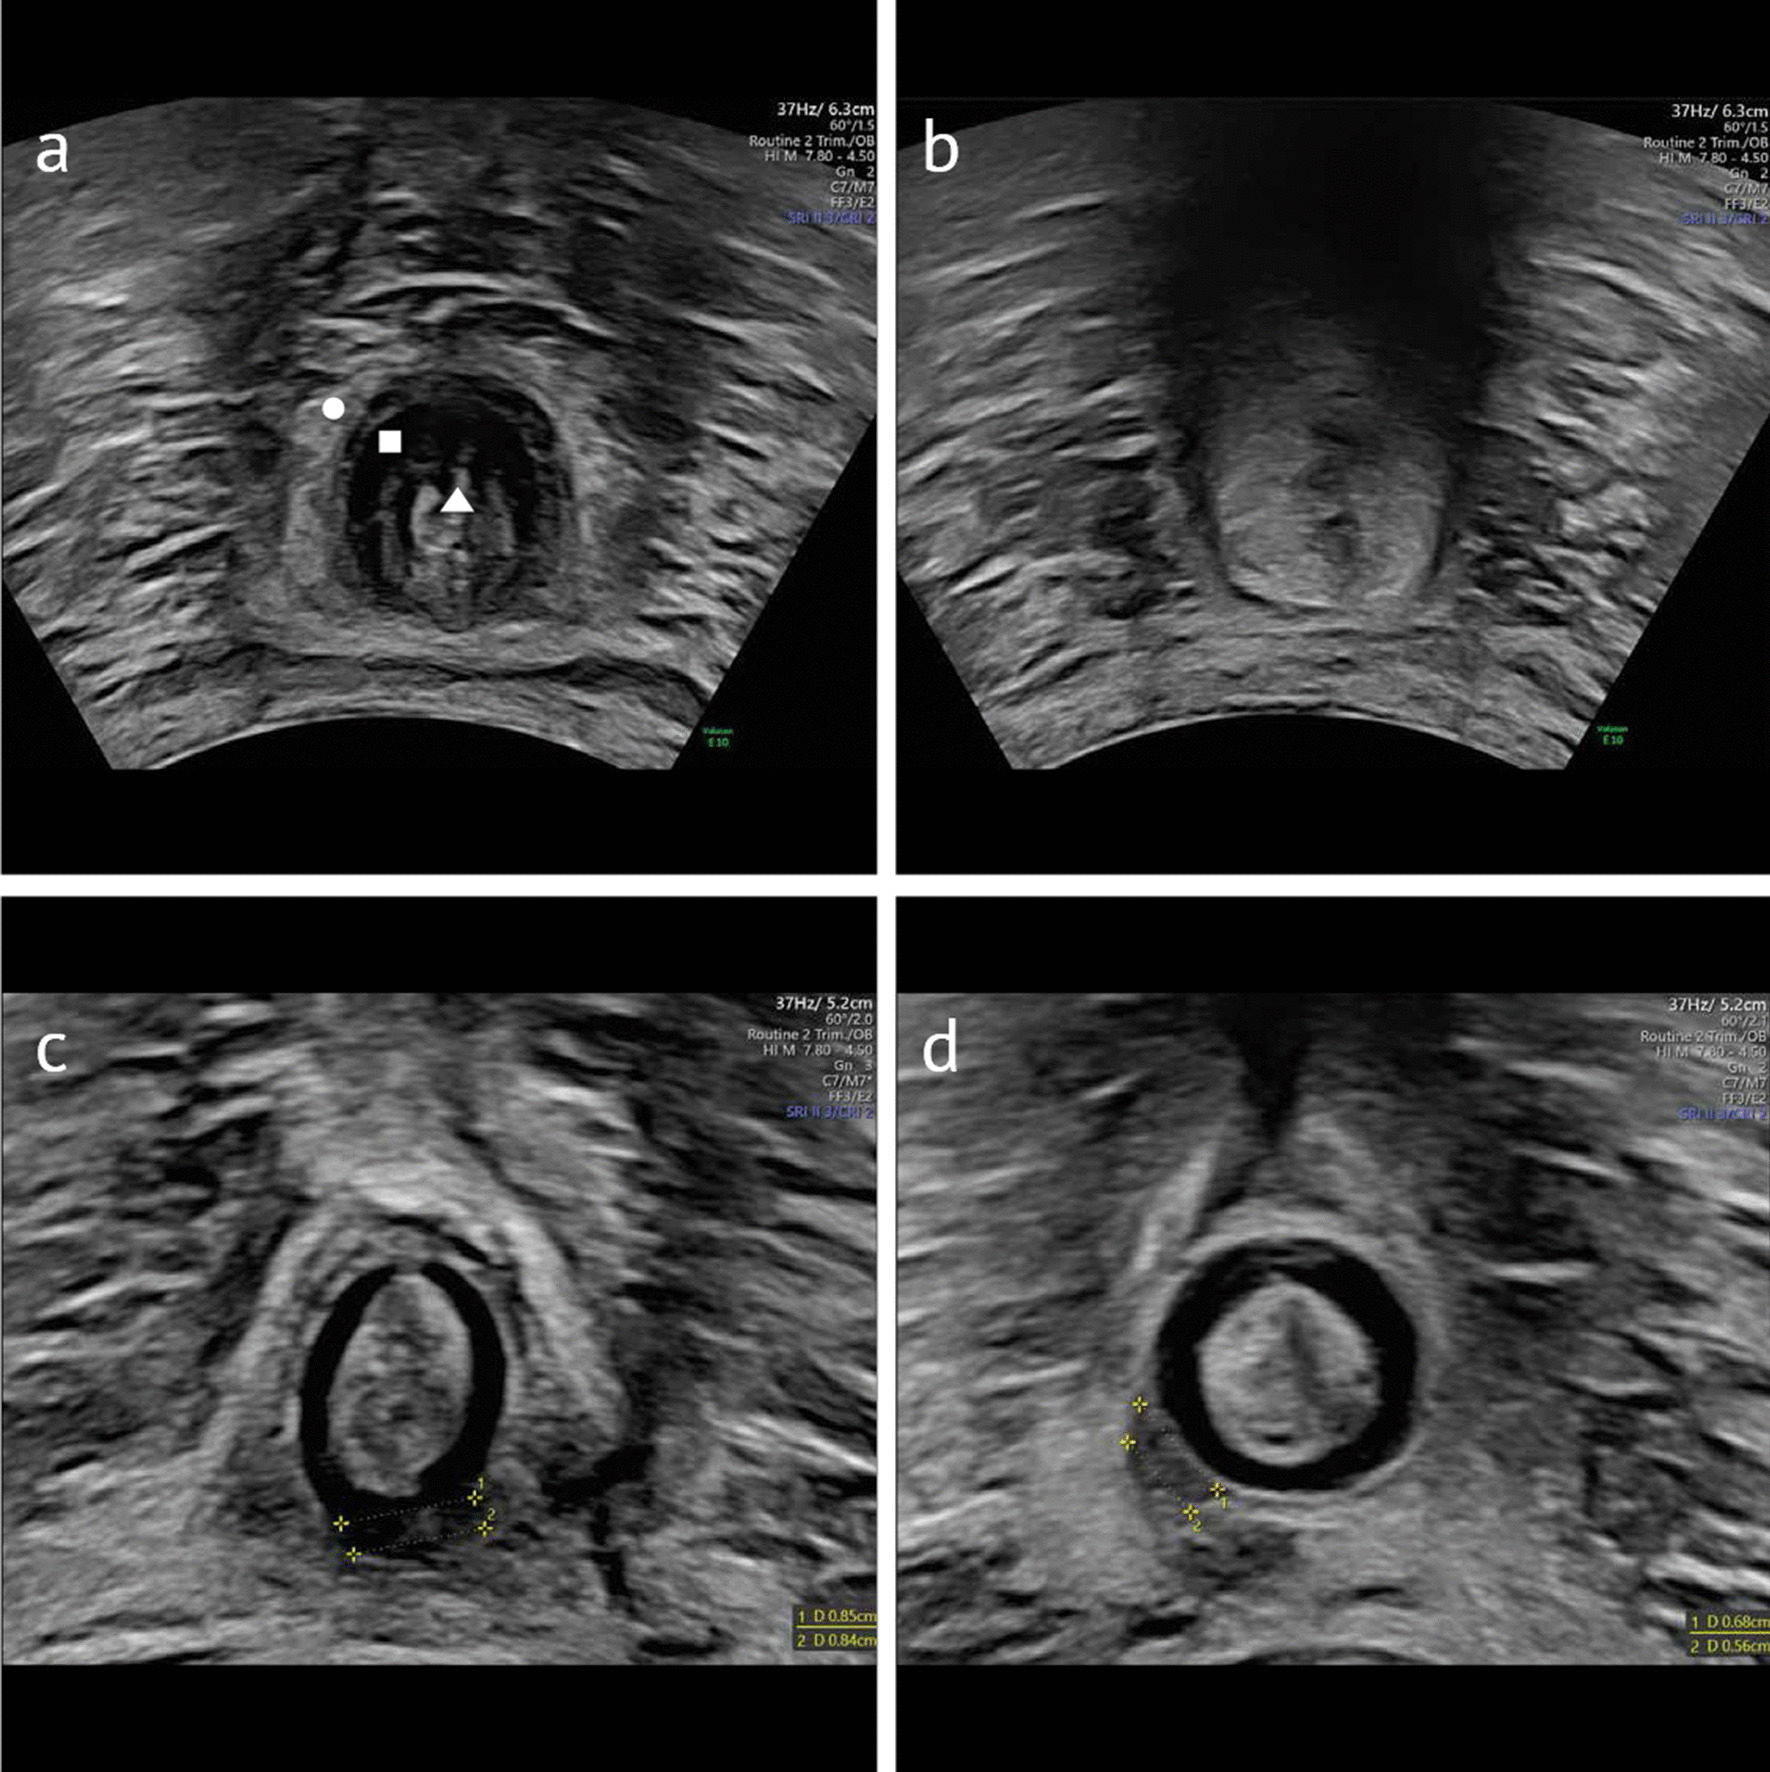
Transperineal ultrasound of the anal sphincter. a Intact anal sphincter EAS IAS lumen b positive contraction effect—the sphincter disappears c complete tear of the EAS 3 days after delivery d scar of the EAS after 6 months
